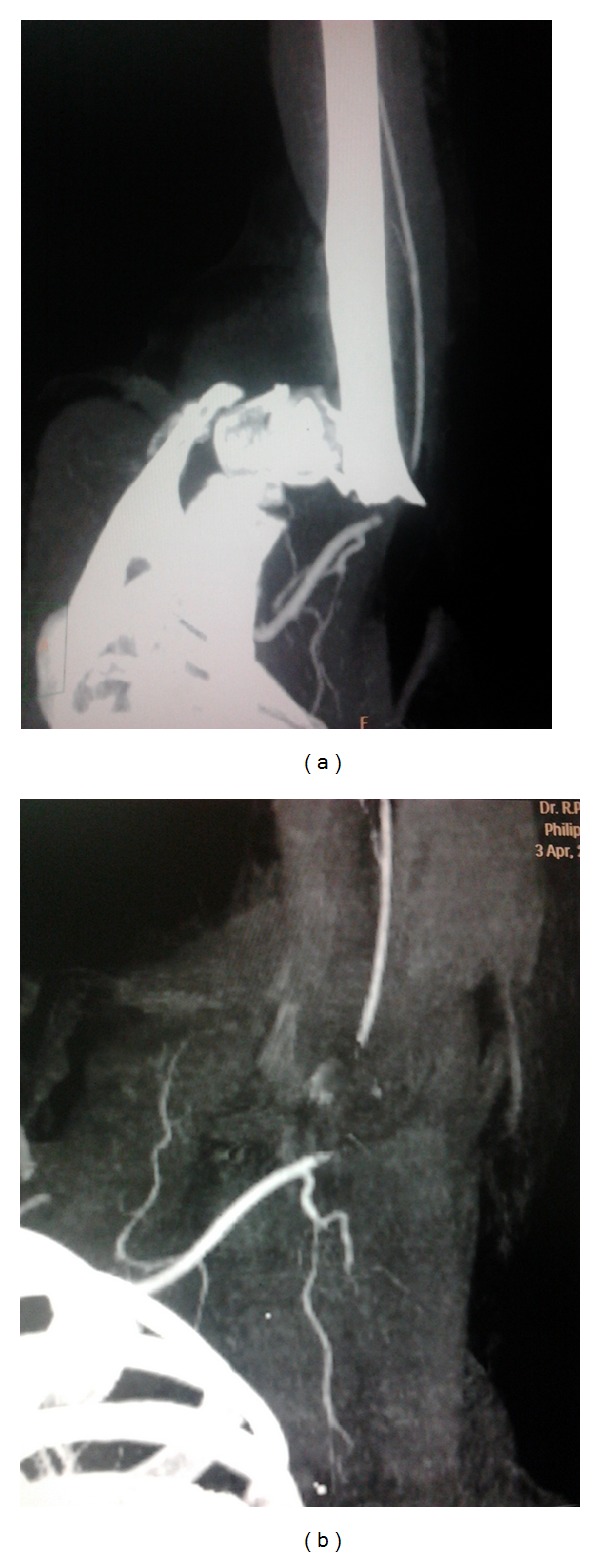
(a) Preoperative CT angiogram showing injury to left axillary artery by fracture proximal end of humerus. (b) Preoperative CT angiogram showing complete occlusion of left axillary artery.
